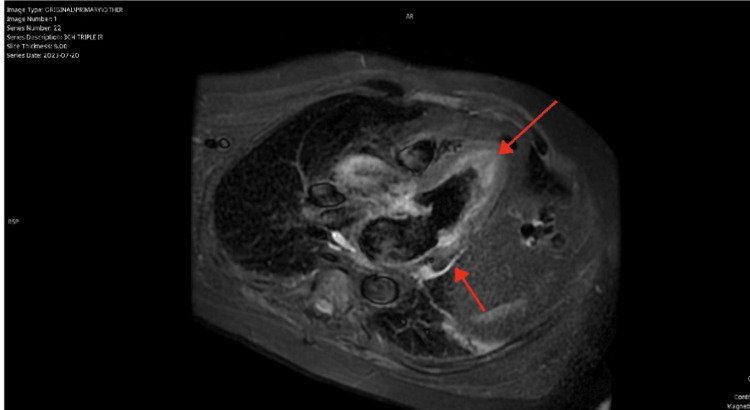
Cardiac MRI (3 chamber triple IR) showing increased myocardial signal intensities in the basal inferolateral and mid-cavity anterolateral segments in keeping with myocarditis (red arrows) IR: Inversion recovery; MRI: Magnetic resonance imaging
